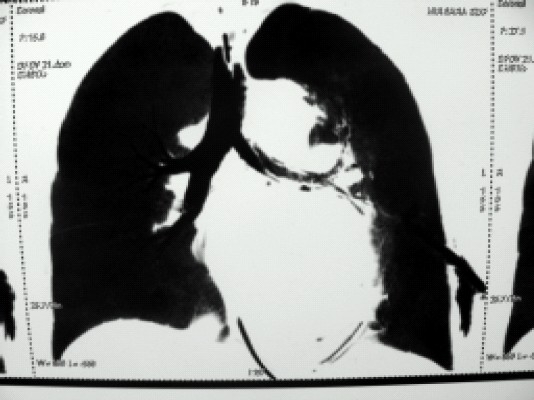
Computed tomography of the chest showing a clot in the left main bronchus arising from trachea (tip of ETT).
